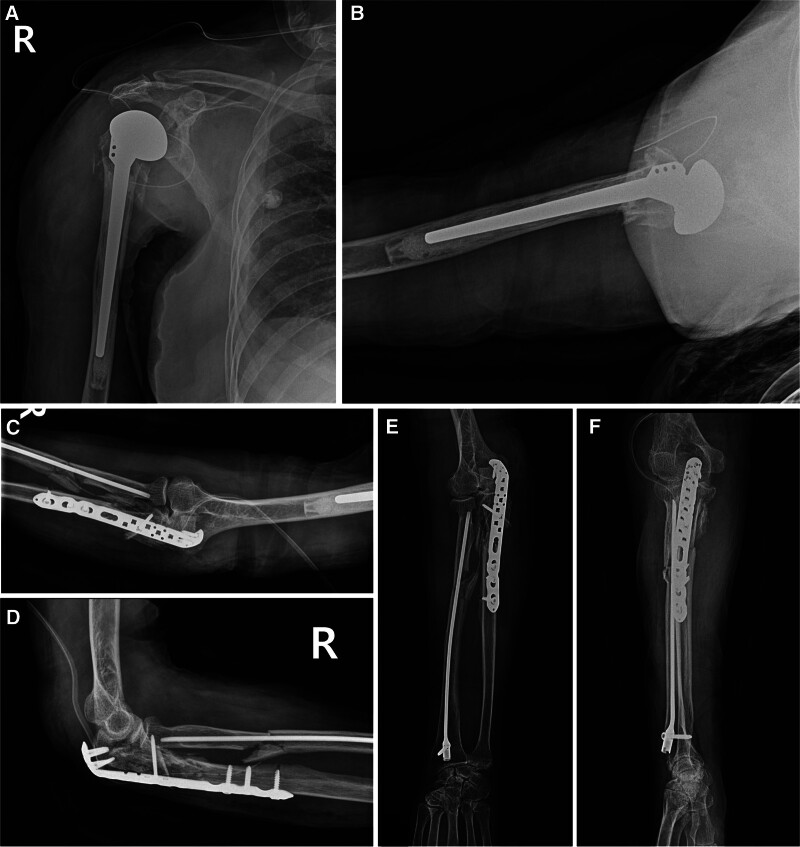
Patient’s immediate post-operative plain radiographs. (A, B) Bipolar hemiarthroplasty for the shoulder fracture dislocation was performed. (C–F) Open reduction and internal fixation were performed for the proximal ulna fracture. However, the skin condition of the anterior side of the forearm was poor due to the open wound at the fracture site, and to shorten the surgery time, intramedullary nail fixation was performed for the proximal radius fracture.
